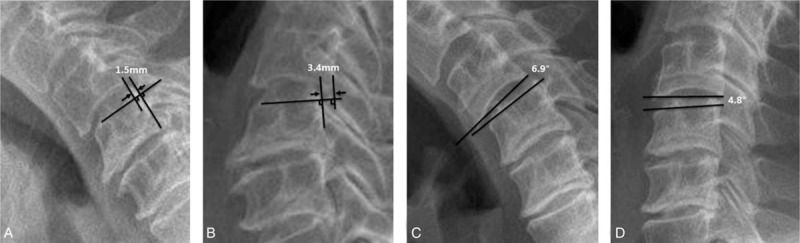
Identification of cervical instability. The slippage displacement of an adjacent vertebra is 4.9 mm (1.5 + 3.4 mm) > 3.5 mm on flexion/extension X-ray radiographs (A, C) or the segmental ROM is 11.7° (6.9° + 4.8°) > 11° (C, D) would be judged as cervical instability.
